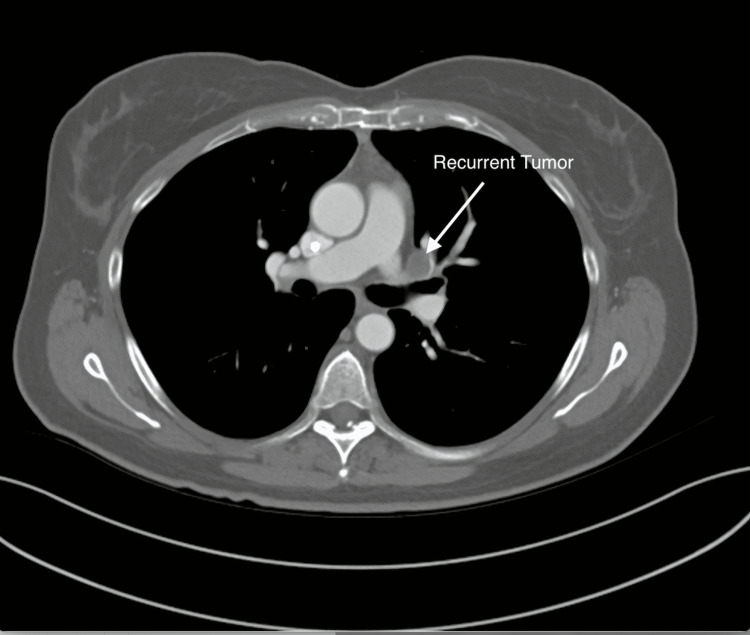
Follow-up CT scan on 3/5/19, demonstrating a new 1.4 cm filling defect in the left upper lobe pulmonary vein.
